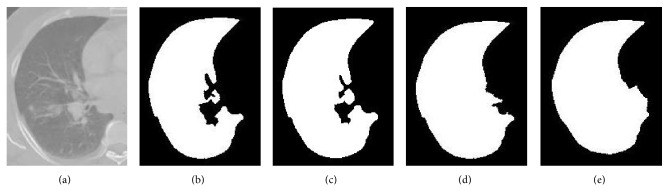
Effects of different neighborhoods used in Section 2.2.5, Stage 2, Step 3: (a) original CT slice, (b) segmented right lung, (c) propagated right lung using 5 × 5 × 5 neighborhood, (d) propagated right lung using 7 × 7 × 7 neighborhood, and (e) propagated right lung using 9 × 9 × 9 neighborhood. Using 7 × 7 × 7 neighborhood, excluded pathological areas are included into the lungs successfully.
